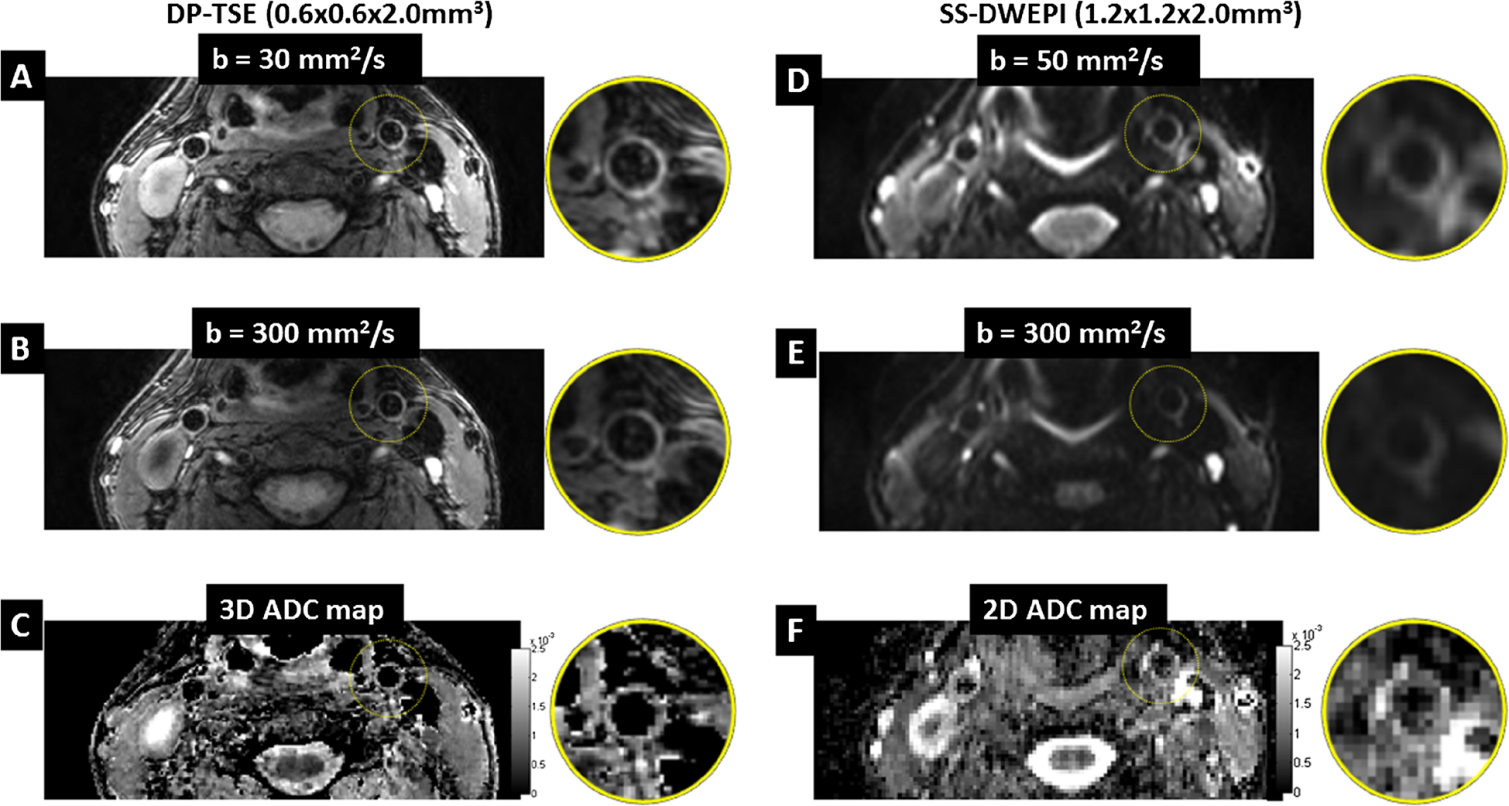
Representative image quality of 3D DP-TSE compared with that of conventional 2D SS-DWEPI from a healthy subject. (A to C) DWI of b = 30 mm^2^/s, DWI of b = 300 mm^2^/s and ADC map, respectively, acquired using DP-TSE with resolution of 0.6 × 0.6 × 2.0 mm^3^. (D to F) DWI of b = 50 mm^2^/s, DWI of b = 300 mm^2^/s and ADC map, respectively, acquired using SS-DWEPI with resolution of 1.2 × 1.2 × 2.0 mm^3^. Note that blurring, distortion and artifacts were present in the SS-DWEPI images compared with their counterparts from DP-TSE. Also note that SS-DWEPI images had higher T2 decay due to the longer required TE than that of the proposed DP-TSE.
